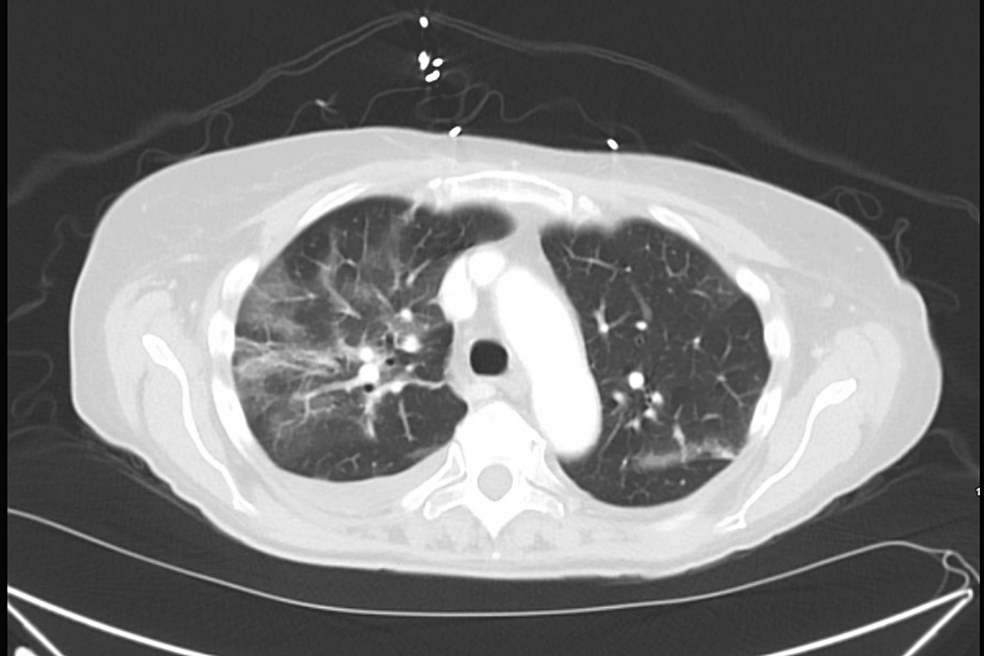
Lung CT scan showing ground-glass opacities throughout the lungs
